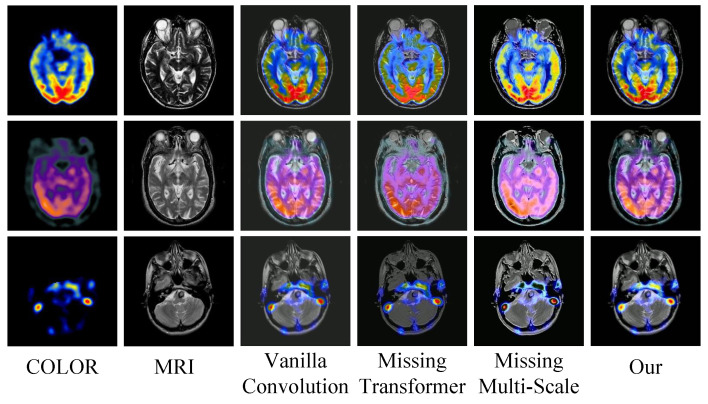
Fusion results under different loss constraints. From left to right: COLOR and MRI images, vanilla convolution instead of ordinary convolution, missing transformer, missing multi-scale, and MDC-RHT.
